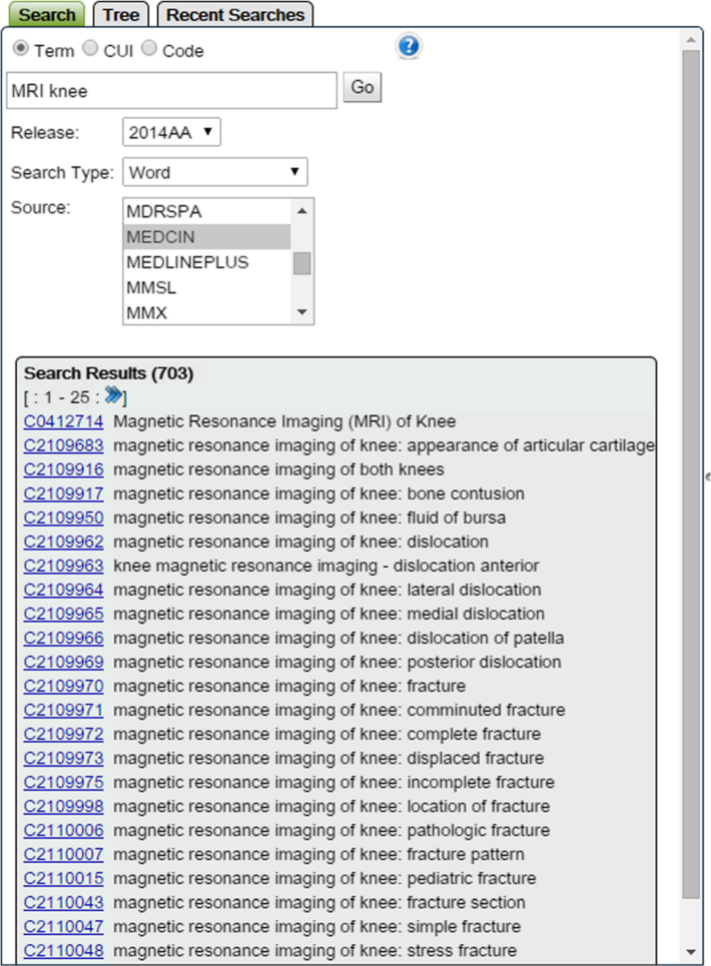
MEDCIN terminology related to MRI of knee. UMLS terminology services were used to access relevant terminology
